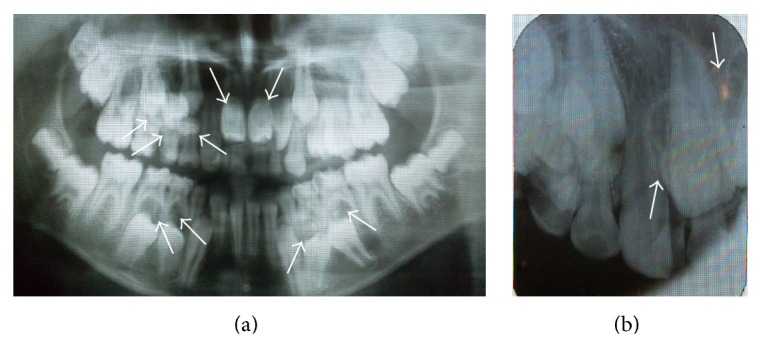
Preoperative radiographs showing multiple supernumerary teeth. (a) The arrows show 9 supernumerary teeth in both jaws. (b) The arrows show 2 supernumerary teeth in the upper anterior region.
